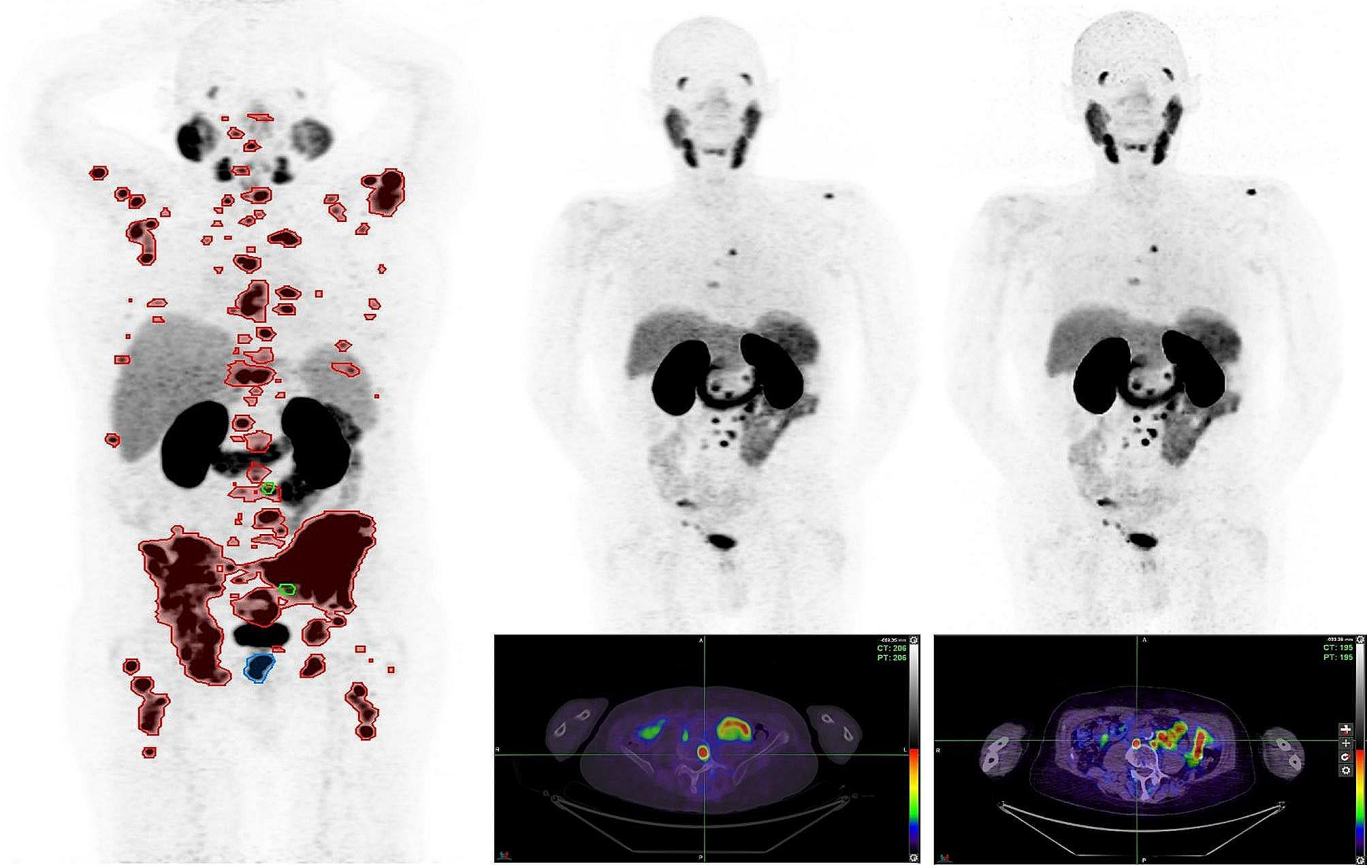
Images quantification (left column) in a patient with local recurrence in prostate bed (blue couture), regional and distant nodal (green contours) and widespread osseous metastatic disease (red contours) with same target lesion (lower thoracic spine) on both OSEM and Q.Clear images. The OSEM (middle column) and BPL (right column) reconstructed images in a different patient, show different target lesions, an osseous lesion (SUVmax: 21.1) on OSEM and a retroperitoneal lymph node (SUVmax: 30.7) on Q.Clear images. The SUVmean and MTV were 6.5 and 55 mL on OSEM compared to 7.1 and 48mL on Q.Clear reconstructed images, respectively
